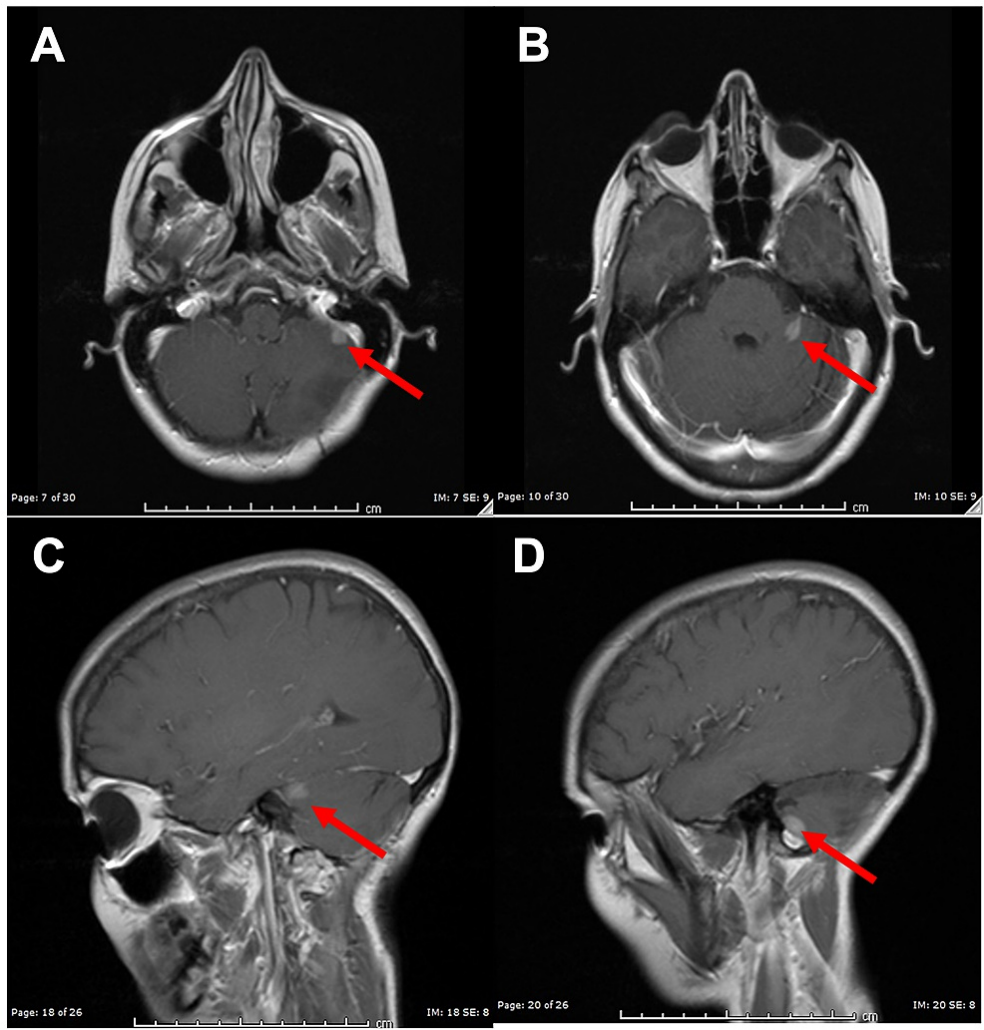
MR Images Following First Dose of Stereotactic Radiosurgery (A, B) Axial T2-weighted MR images demonstrating new enhancing lesions within the left cerebellum and resolution of the original mass after resection (red arrow). (C, D) Sagittal T1-weighted MR images with gadolinium contrast demonstrating new enhancing lesions within the left cerebellum and resolution of the original mass after resection (red arrow).
